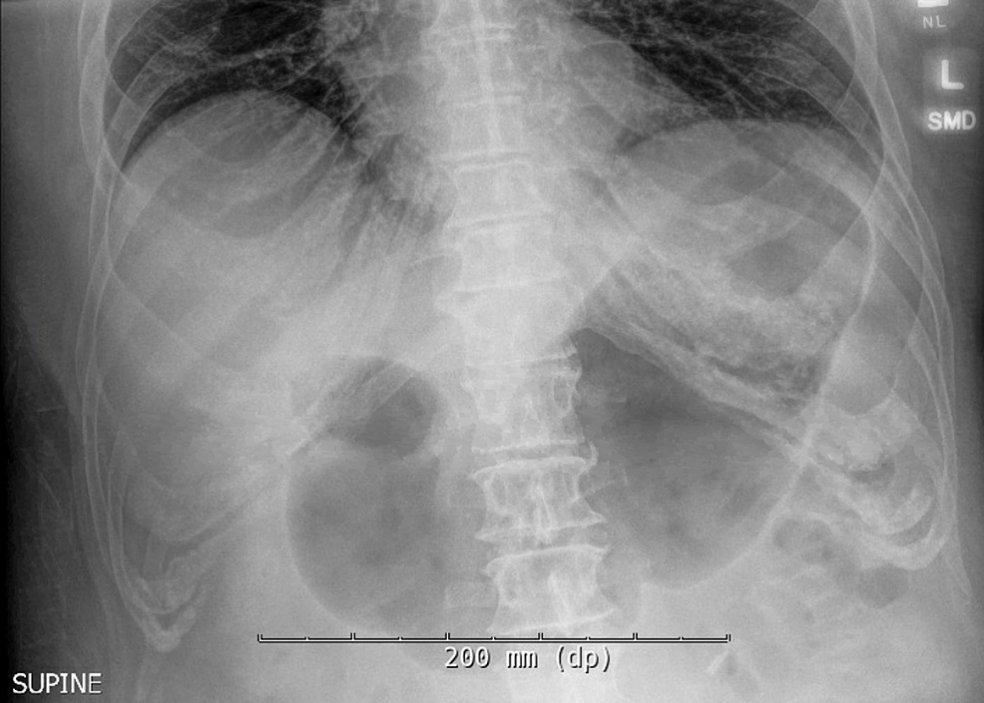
Abdominal x-ray demonstrating gastric distension.
